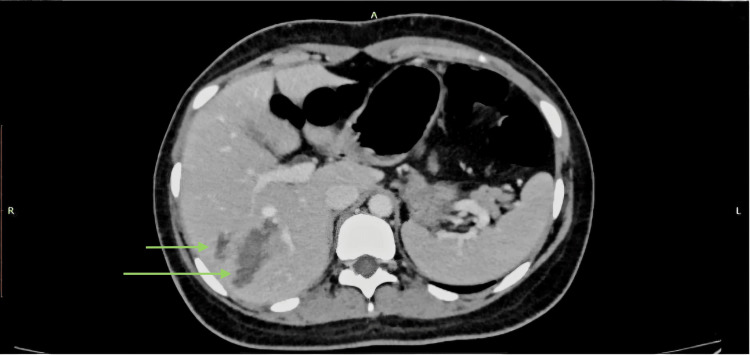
AAST grade II liver injury with linear intraparenchymal lacerations on CECT (PV protocol). A 20-year-old female with a history of RTA underwent CECT of the abdomen using the PV phase. The axial CECT image shows two linear intraparenchymal lacerations involving segments VI and VIII (green arrows) without active contrast extravasation. Findings were consistent with the AAST grade II liver injury. AAST: American Association for the Surgery of Trauma; CECT: contrast-enhanced computed tomography; PV: portal venous; RTA: road traffic accident.
